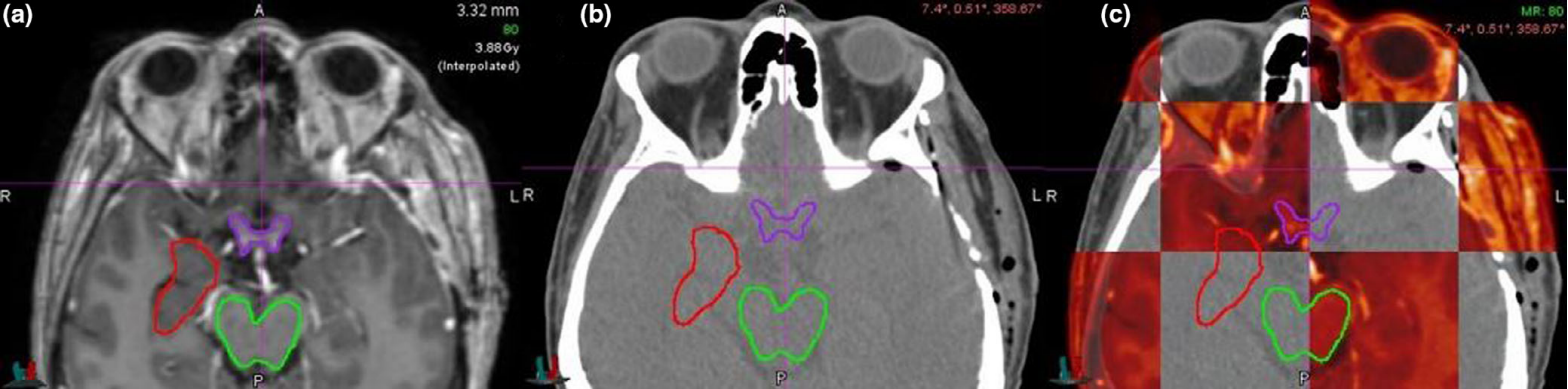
Axial slice of a representative patient showing the right epilepsy target, brainstem, and OA in (A) MRI, (B) CT, and (C) checkerboard pattern of fused MRI with CT image. MRI, magnetic resonance images; OA, optic apparatus.
